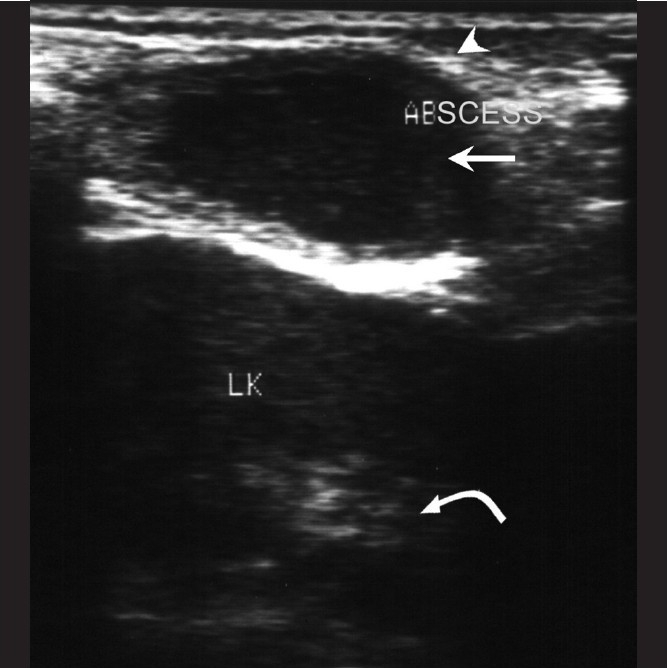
Rib tuberculosis – patient 12. Ultrasonography of the lower chest wall shows a hypoechoic collection (arrow) with echogenic walls (arrow head) overlying the left kidney (curved arrow)
